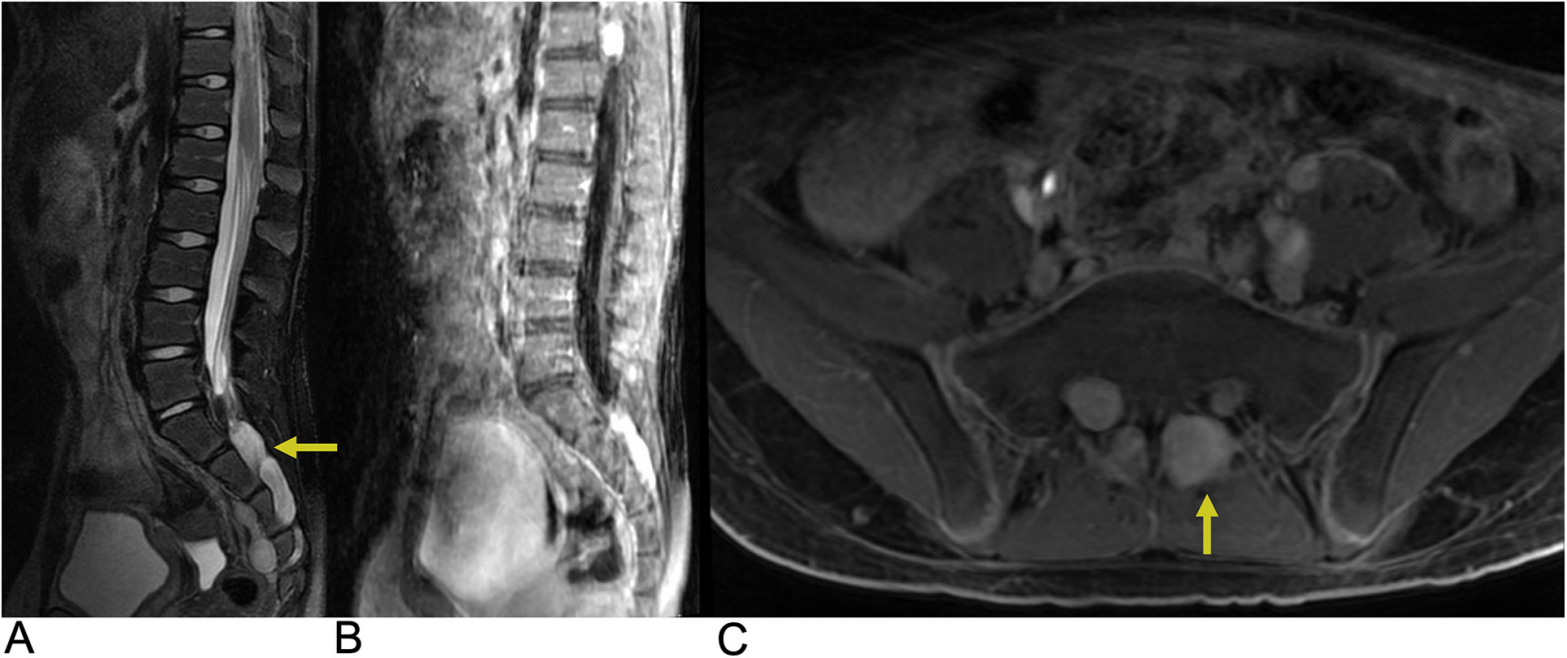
Neurofibroma. Sagittal T2 FLAIR (A), sagittal T1 FLAIR + C (B), and axial T1 FAT + C (C) MRI demonstrating numerous neurofibromas (yellow arrows) throughout the lumbosacral spine with involvement of the L3–L4 and S1–S4 levels along the greater sciatic notch with associated remodeling of the neural foramina.
